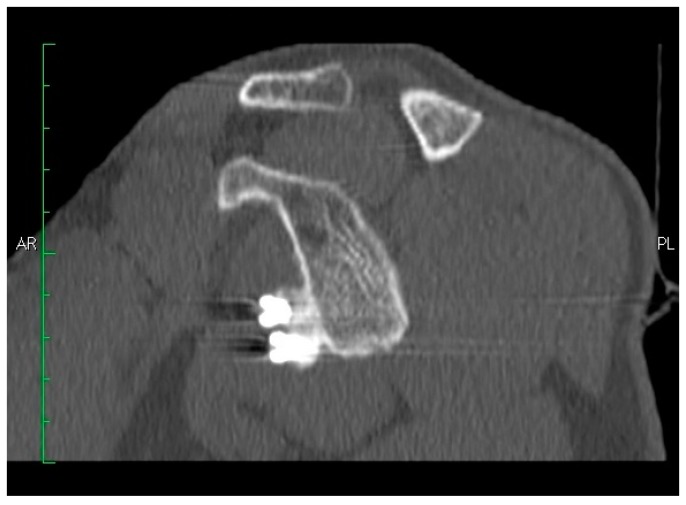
Sagittal computed tomography scan of a united coracoid graft.
